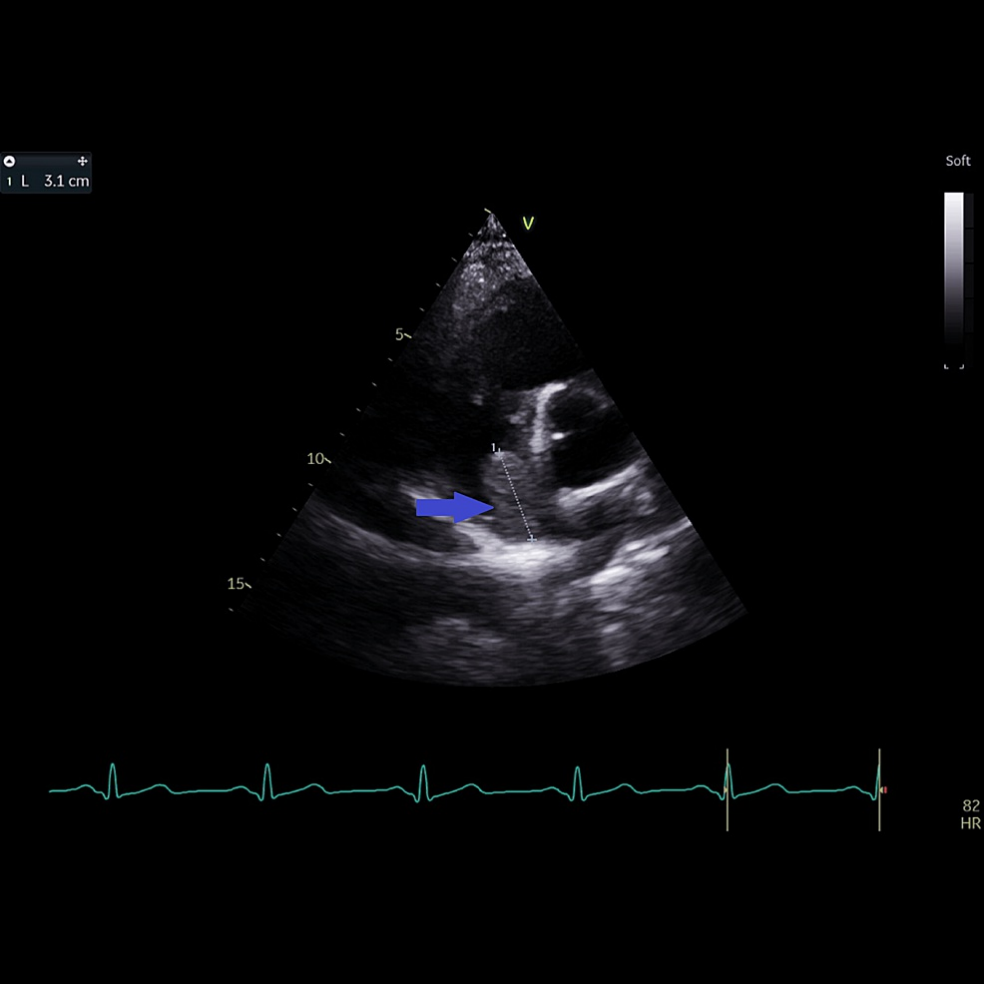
Modified short-axis view at the base of the heart from the left parasternal window showing the right atrial mass (blue arrow); but the origin or the attachment of the mass could not be visualized from this view. The lower (juxta-atrial) superior vena cava could not be visualized from this view.
